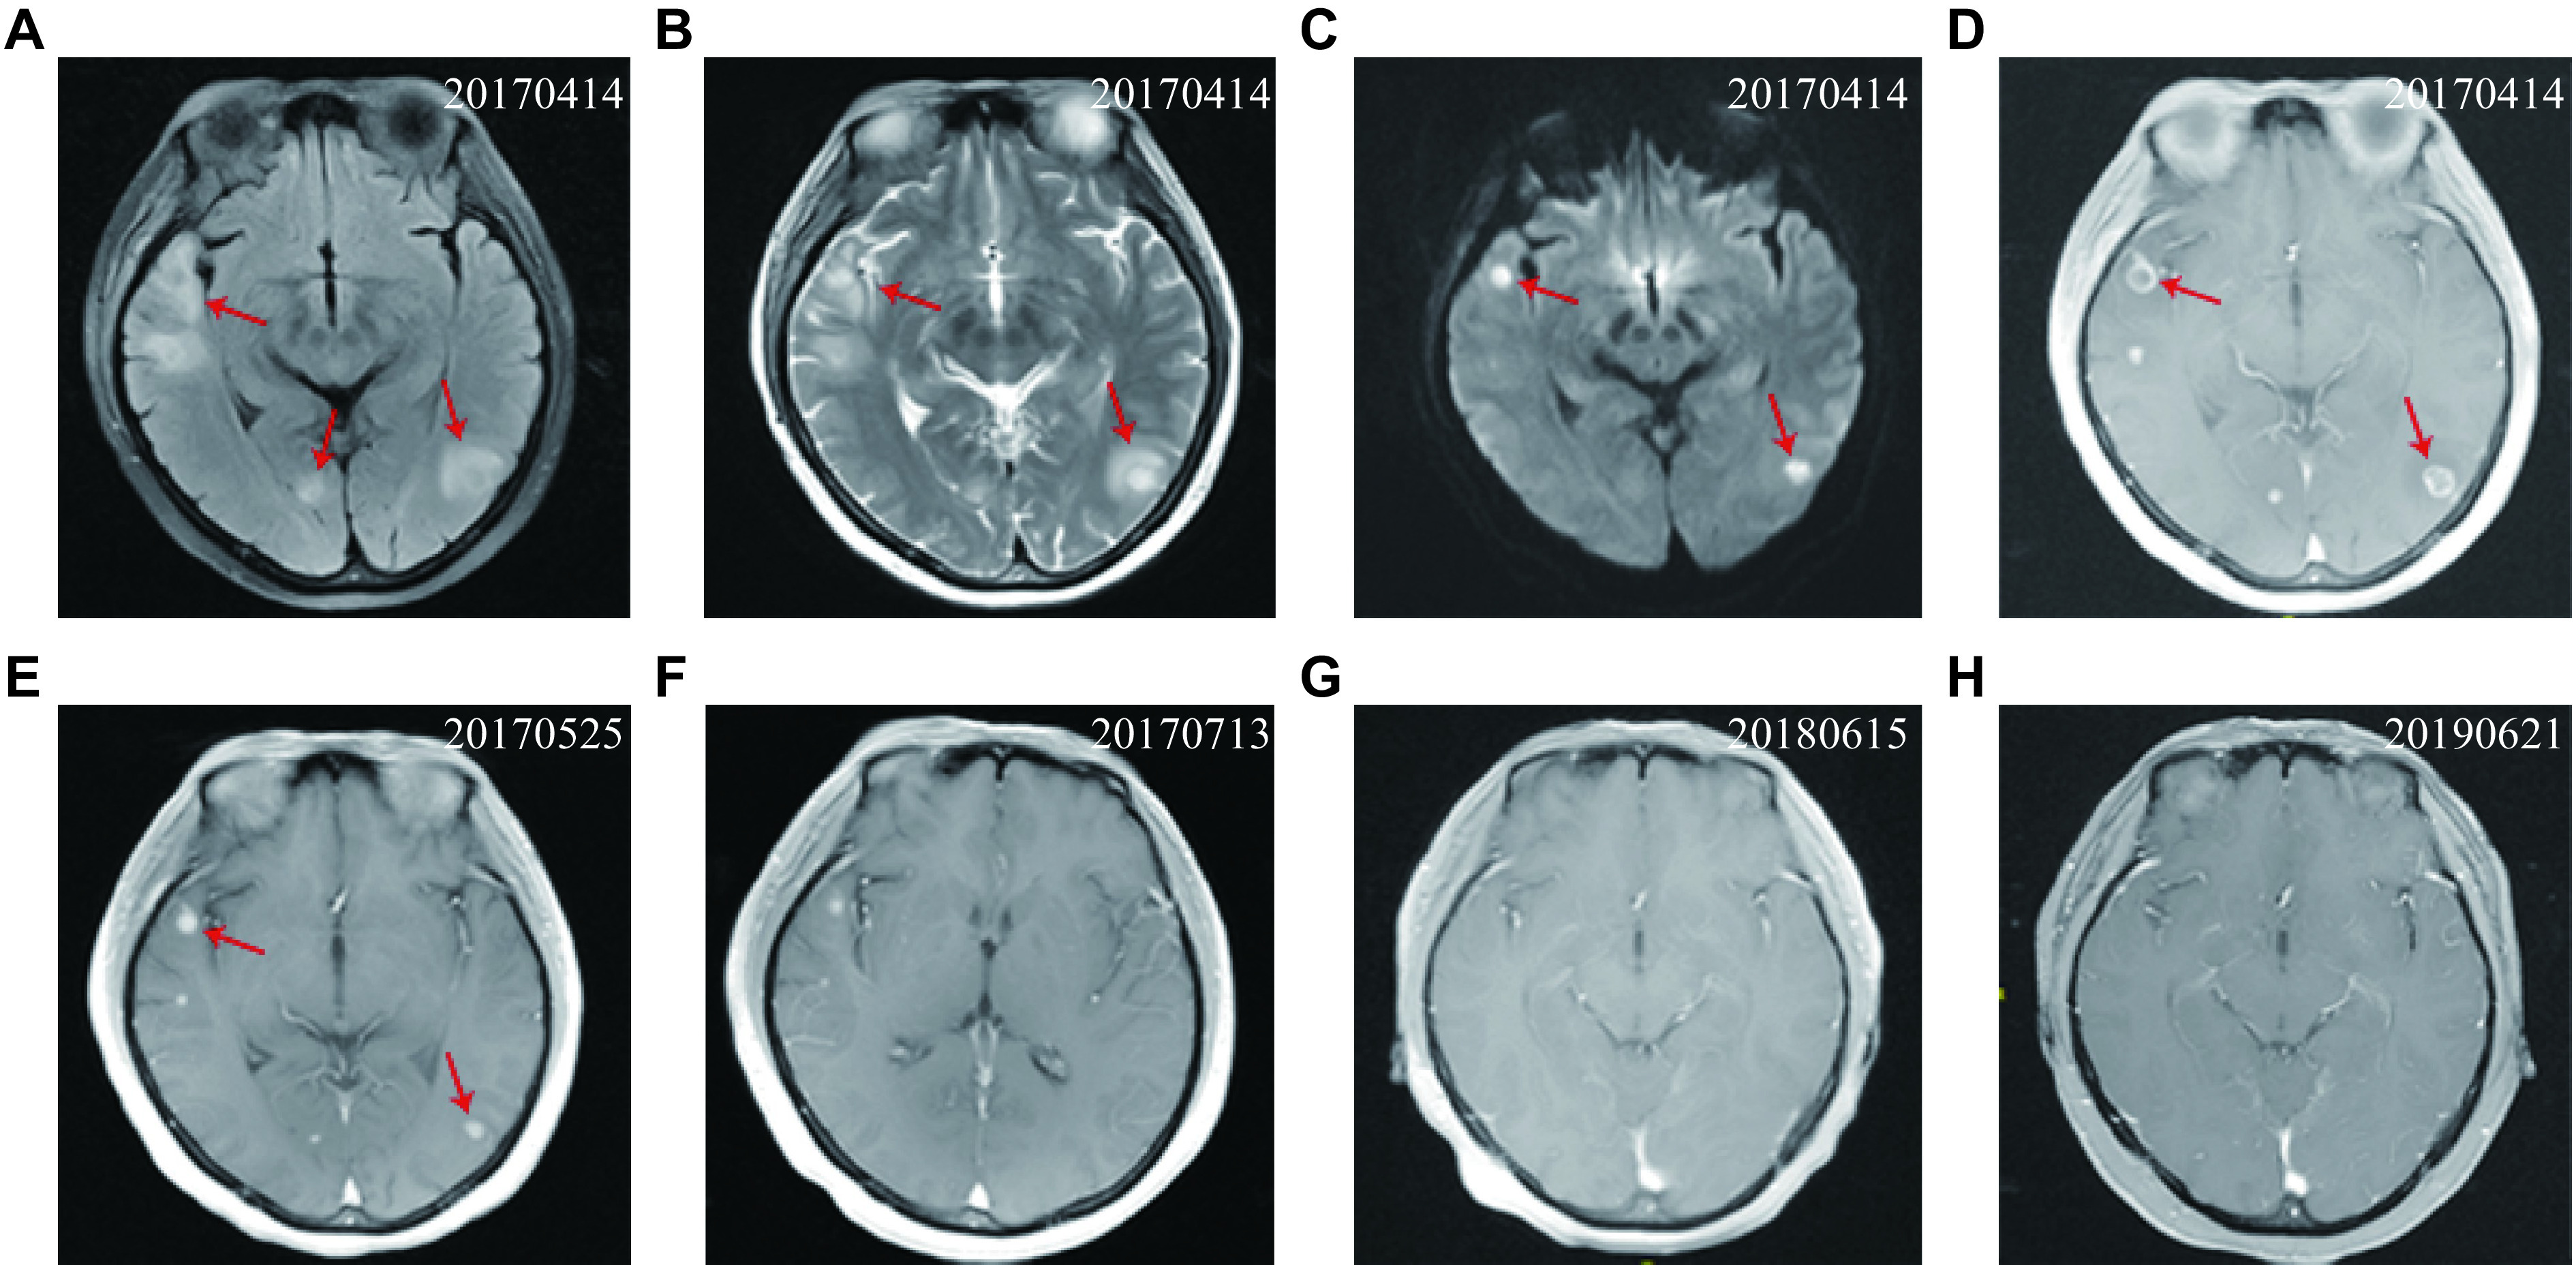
Magnetic resonance imaging (MRI) views of the brain abscess before and during the follow-up.
